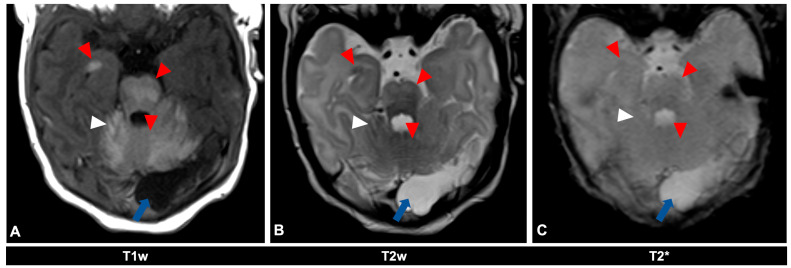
MR imaging of an 11-day-old female patient with neurocutaneous melanosis (NCM) using an MRI-compatible incubator. (A–C) Imaging findings of leptomeningeal (white arrowhead) and intraparenchymal melanosis (red arrowhead) demonstrate a high T1 signal, especially in the pons, cerebellum, and amygdala. These findings are not sufficiently detectable in T2-weighted (B) or T2 star (C) sequences (white arrowhead), at most a slight signal reduction in T2-weighted sequences. The patient also has an infratentorial arachnoid cyst (blue arrow). Spinal MRI showed no abnormalities (not shown). T1w = T1-weighted; T2w = T2-weighted; T2* = T2 star.
